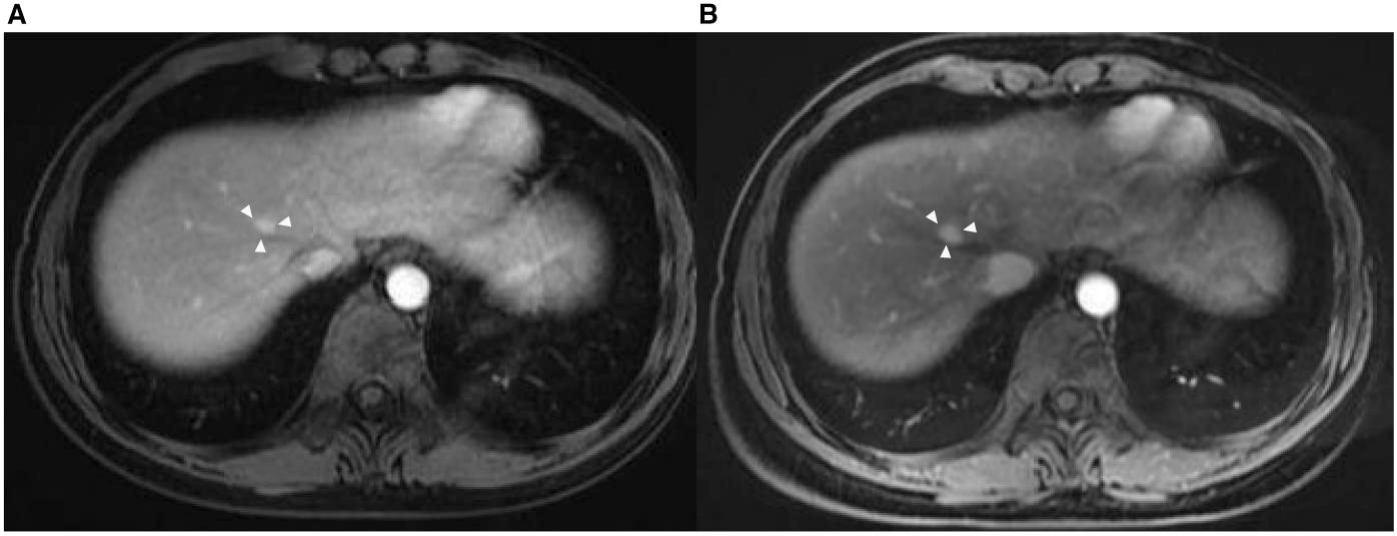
Liver metastasis during follow-up. (A) MRI image at 34 months postsurgery showing a small liver metastasis (white arrowheads). (B) Follow-up MRI at 38 months showing enlargement of the lesion prior to intervention with radiofrequency ablation and combination chemotherapy (white arrowheads). Abbreviation: MRI, magnetic resonance imaging.
